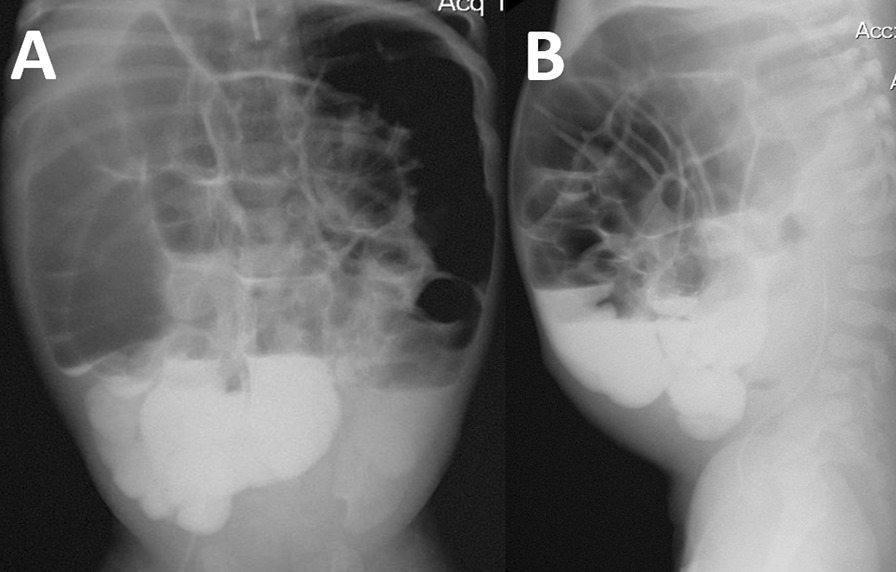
Anteroposterior (A) and lateral (B) oral contrast radiographs depicting Kloiber cups, flatulence, and megacolon (day 75 after hospitalization)
